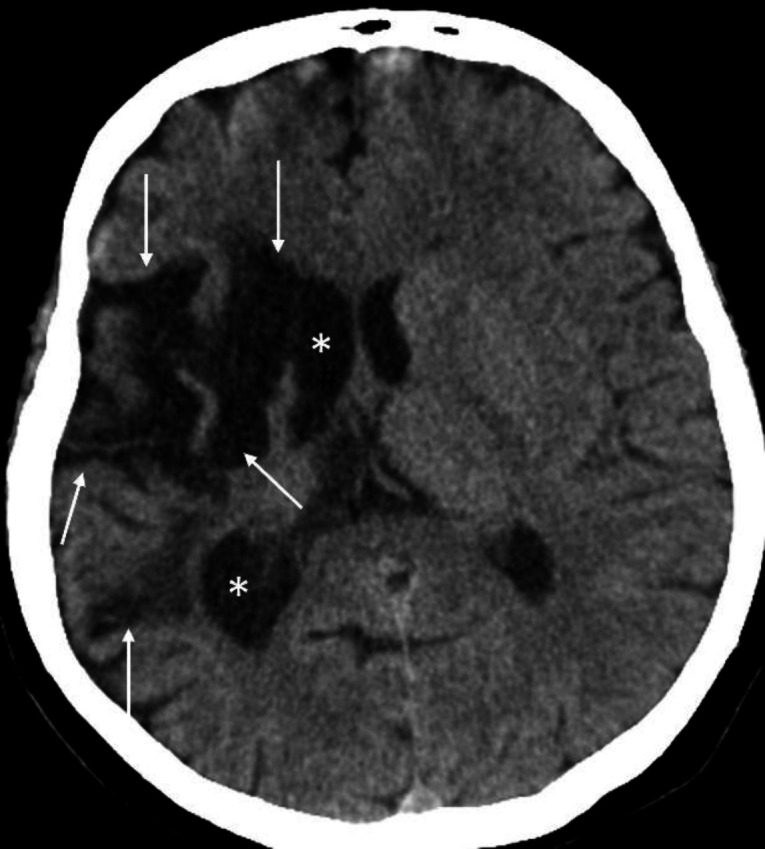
Non-contrast axial CT brain of an 82 years-old female with a history of a prior stroke shows an old infarction in the right middle cerebral artery territory (arrows). Note the dilatation of the ipsilateral ventricle (asterisks) due to loss of brain volume
